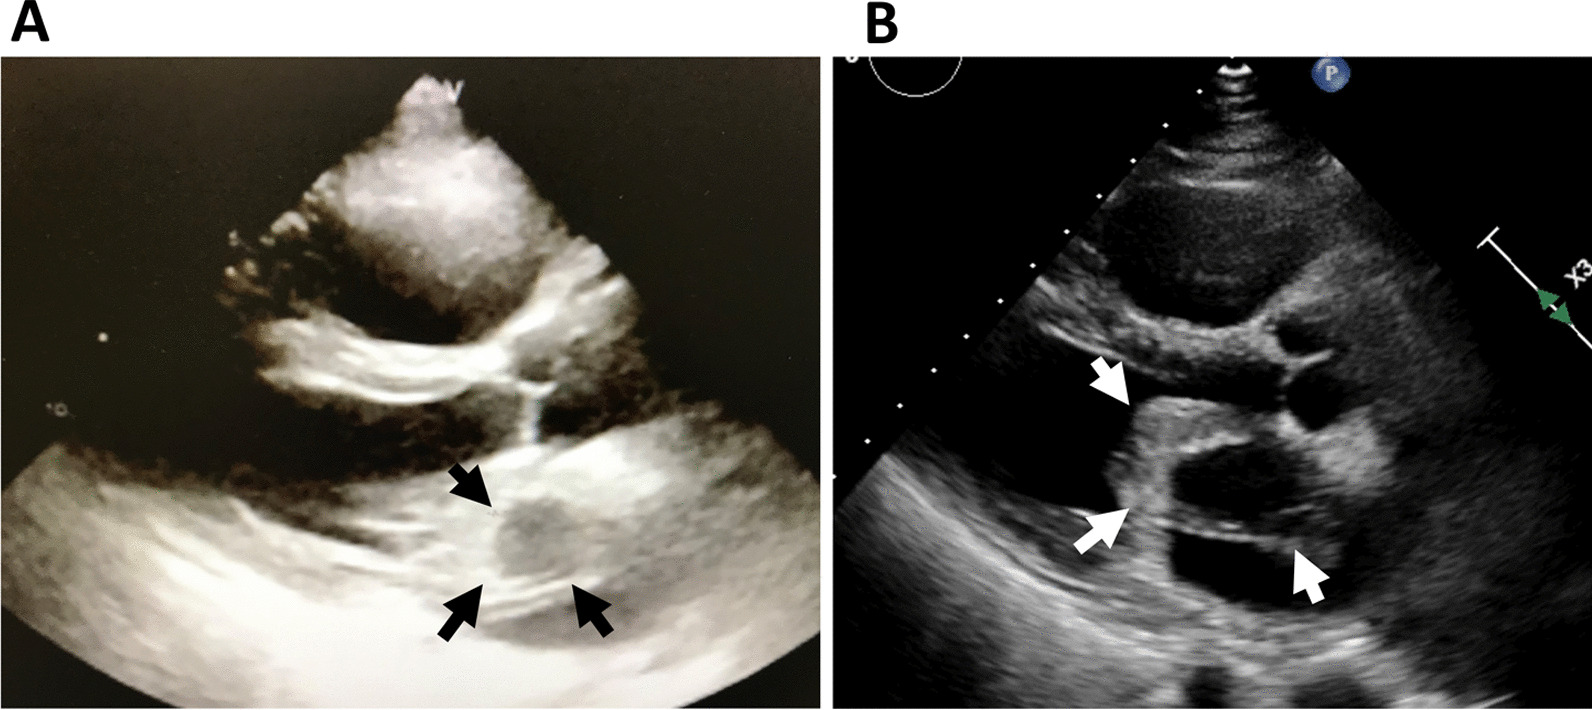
Transthoracic echocardiogram in the parasternal long-axis view. A An image captured by the physician in the primary clinic two hours before admission to our hospital. An oval tumor (arrows) measuring about 25 × 35 mm in the left atrium was revealed. There was a 12-mm round hypoechoic area within the tumor. B An image taken upon admission to our hospital. A tumor (arrows) approximately 40 × 50 mm in size included a 20 × 30-mm-sized cystic lesion within it. The tumor and its internal cystic lesion were obviously expanded compared to those in the image captured in the primary clinic two hours earlier
